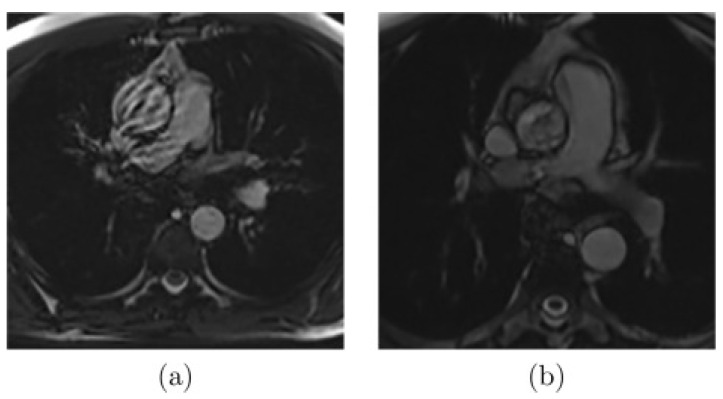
Examples of MR images with artifacts due to (a) rapid or (b) turbulent blood flow [20].
